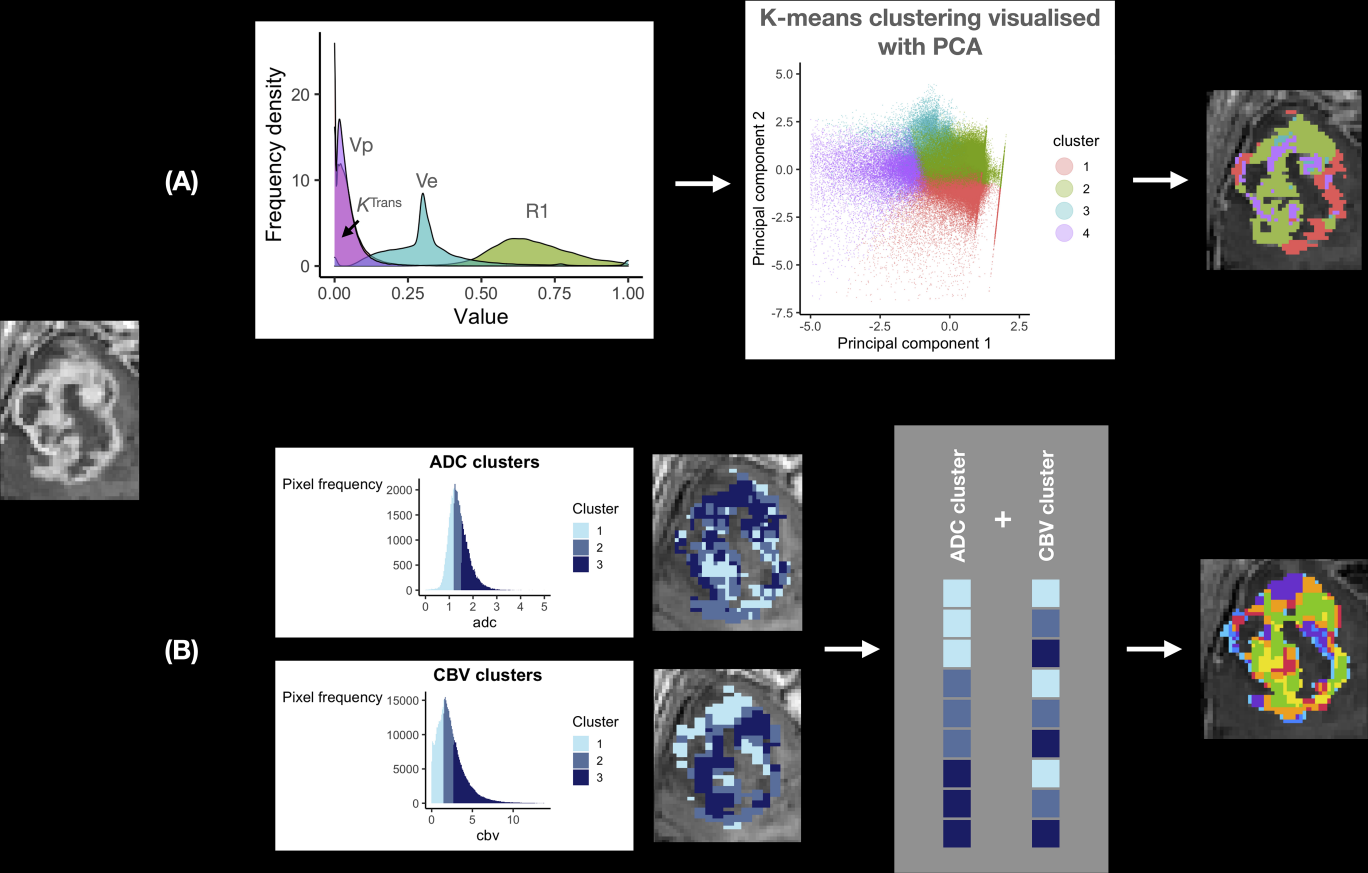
Habitat imaging methods in glioblastoma. This figure provides an overview of the two main approaches to deriving imaging habitats utilising local preoperative data from 12 patients with glioblastoma undergoing surgery. (A) one step approach: a multi-dimensional dataset can be produced utilising multiple imaging biomarkers from the same MRI acquisition (to avoid interpolation/registration errors), in this case Dynamic Contrast Enhanced (DCE) MRI. Data from R1_N_ – defined in Table 1 and three DCE-MRI imaging biomarkers (*K*
